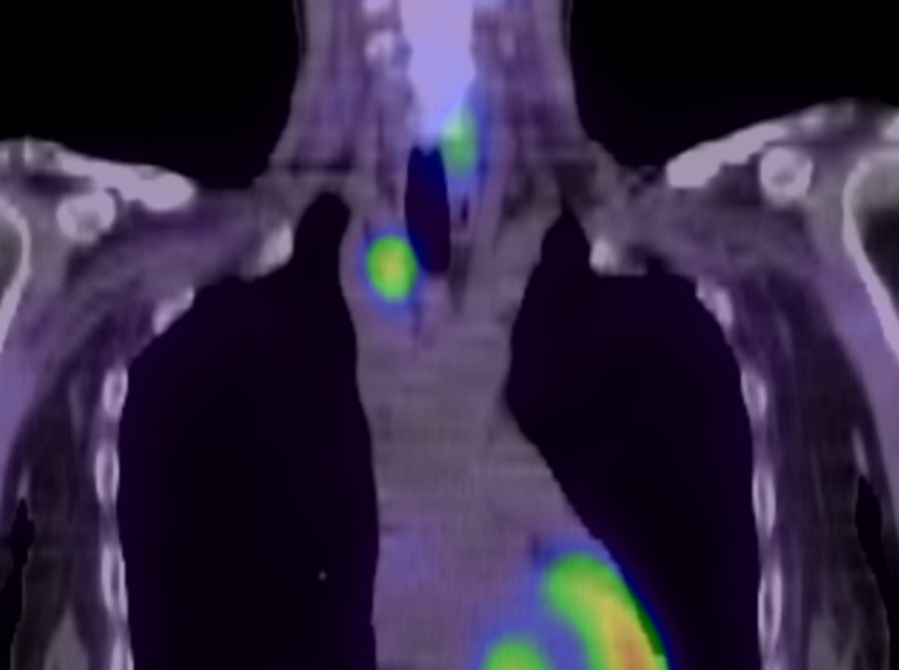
^99m^Tc-methoxyisobutylisonitrile scintigraphy showing increased uptake in the right upper mediastinum after right hemithyroidectomy
